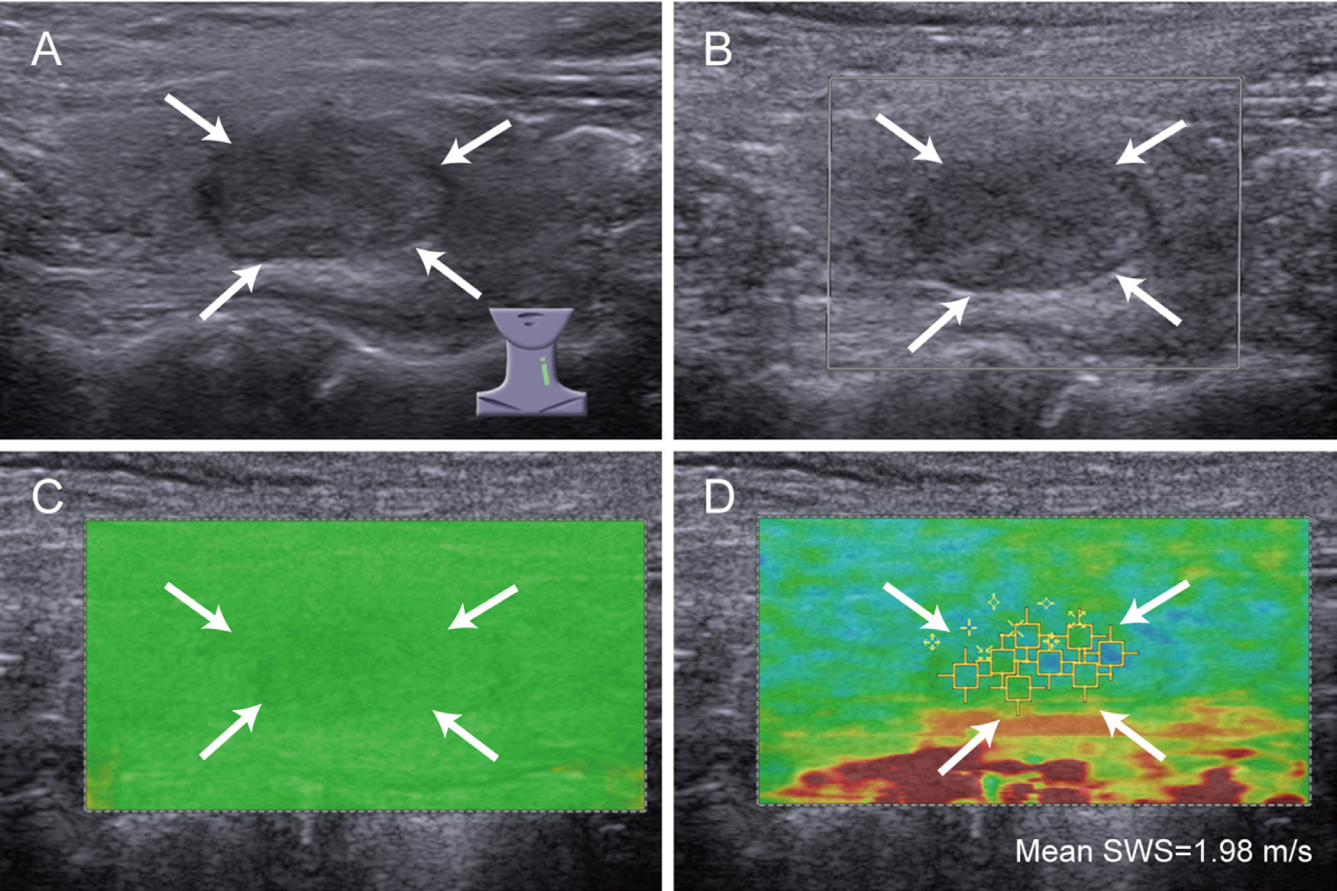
Images of high QM in benign thyroid nodule A 54-year-old woman has nodular goiter. (A) conventional ultrasound shows a 14 mm thyroid nodule (arrows) in left thyroid lobe, which is solid, hypoechoic and regular; (B) color Doppler ultrasound shows no color blood flow signal in the nodule (arrows); (C) SW-quality map shows almost green in the nodule (arrows), indicating high QM; (D) the mean SWS of the nodule (arrows) is 1.98 m/s on SW-velocity map.
